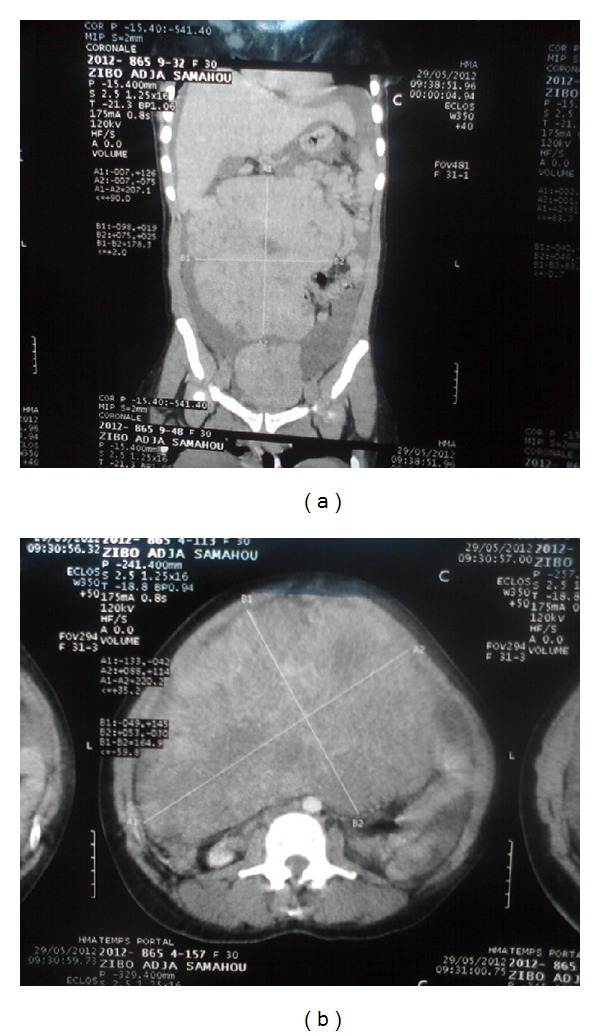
Abdominal CT scan showing two abdominopelvic tissue masses multilobulated.
